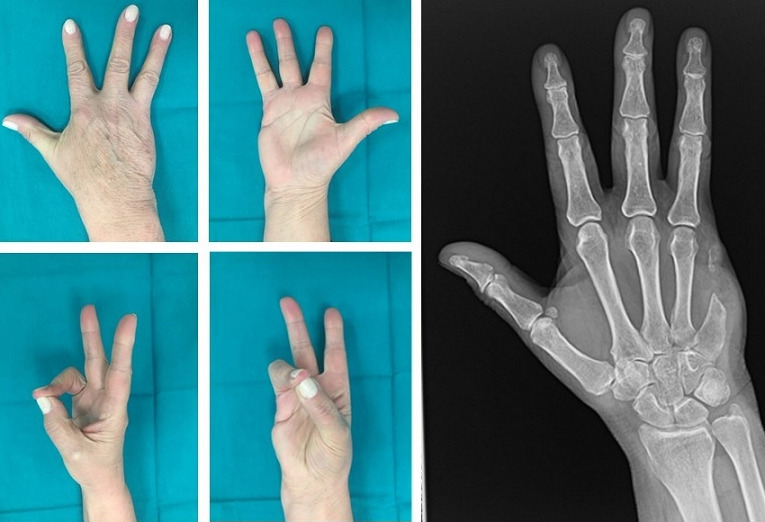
Clinical and radiographic result after one year from surgery
